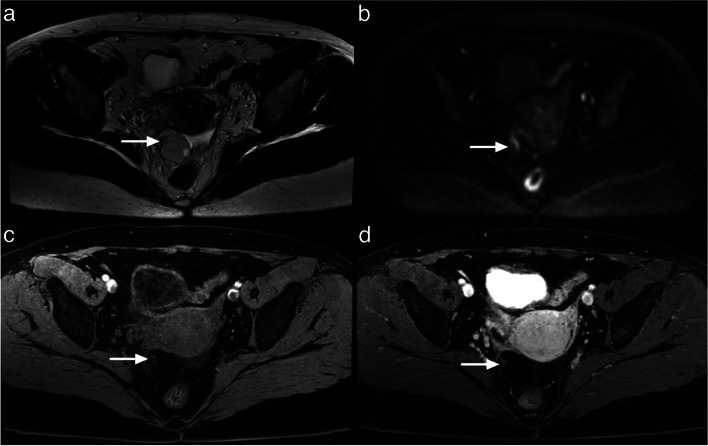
Example of MRI appearance of a Rokitansky nodule which does not account for a solid tissue. a Axial T2-weighted sequence. b Axial DWI abdominal and pelvic scan (*b*: 1200 s/mm^2^). c Axial unenhanced T1-weighted in-phase sequence. d Axial late-gadolinium T1-weighted sequence. Right ovarian dermoïd cyst with a small Rokitansky nodule (white arrow)
